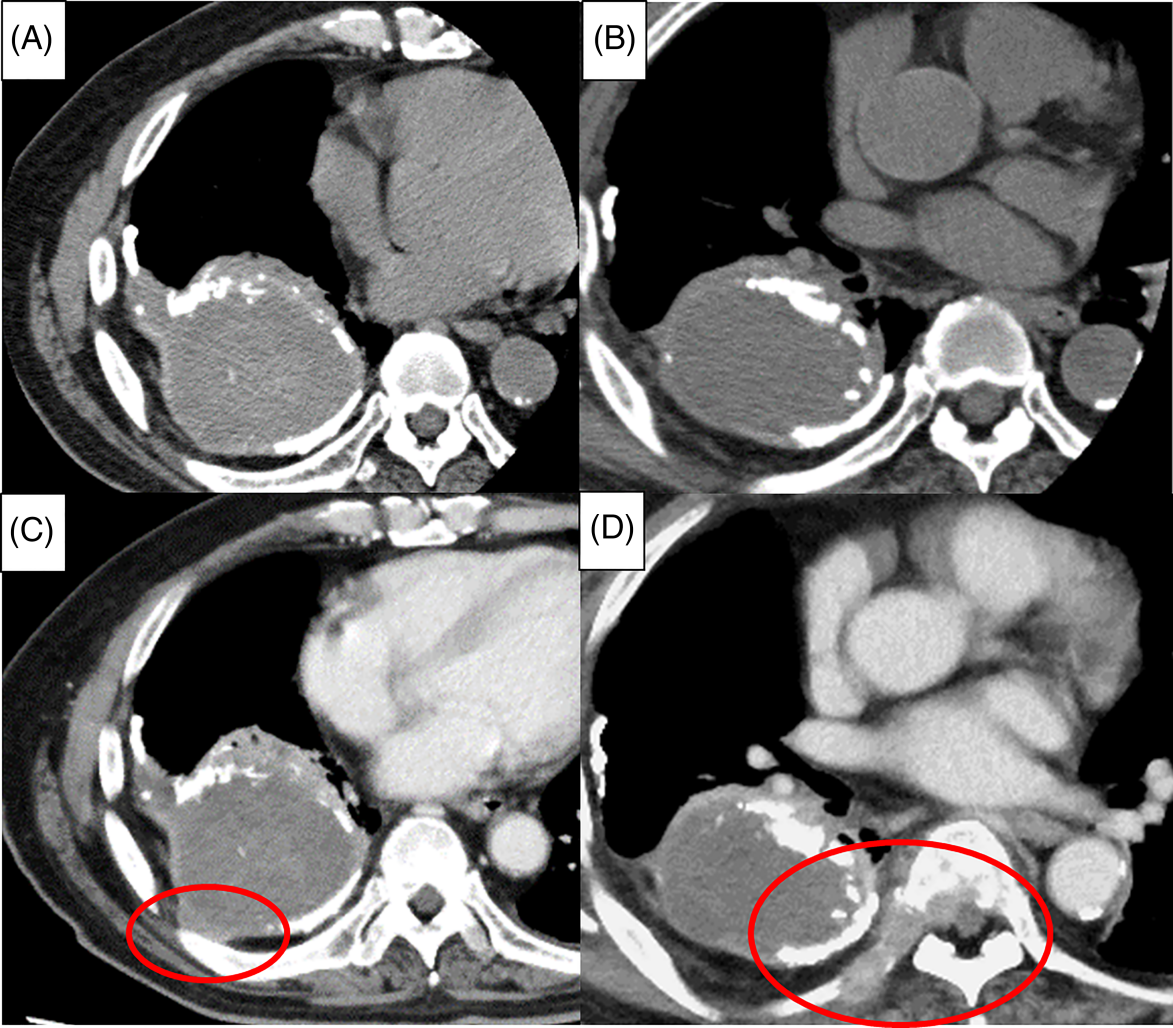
Computed tomography (CT) scan in August 2017. (A, B) Although chronic empyema is also seen in the previous scan, there is no evidence of malignancy. (C, D) A contrast CT scan in mid‐November 2017 revealing the irregular protruding posterior pleural thickening adjacent to the chronic empyema, and an abnormal right seventh costal mass infiltrating the vertebral body.
